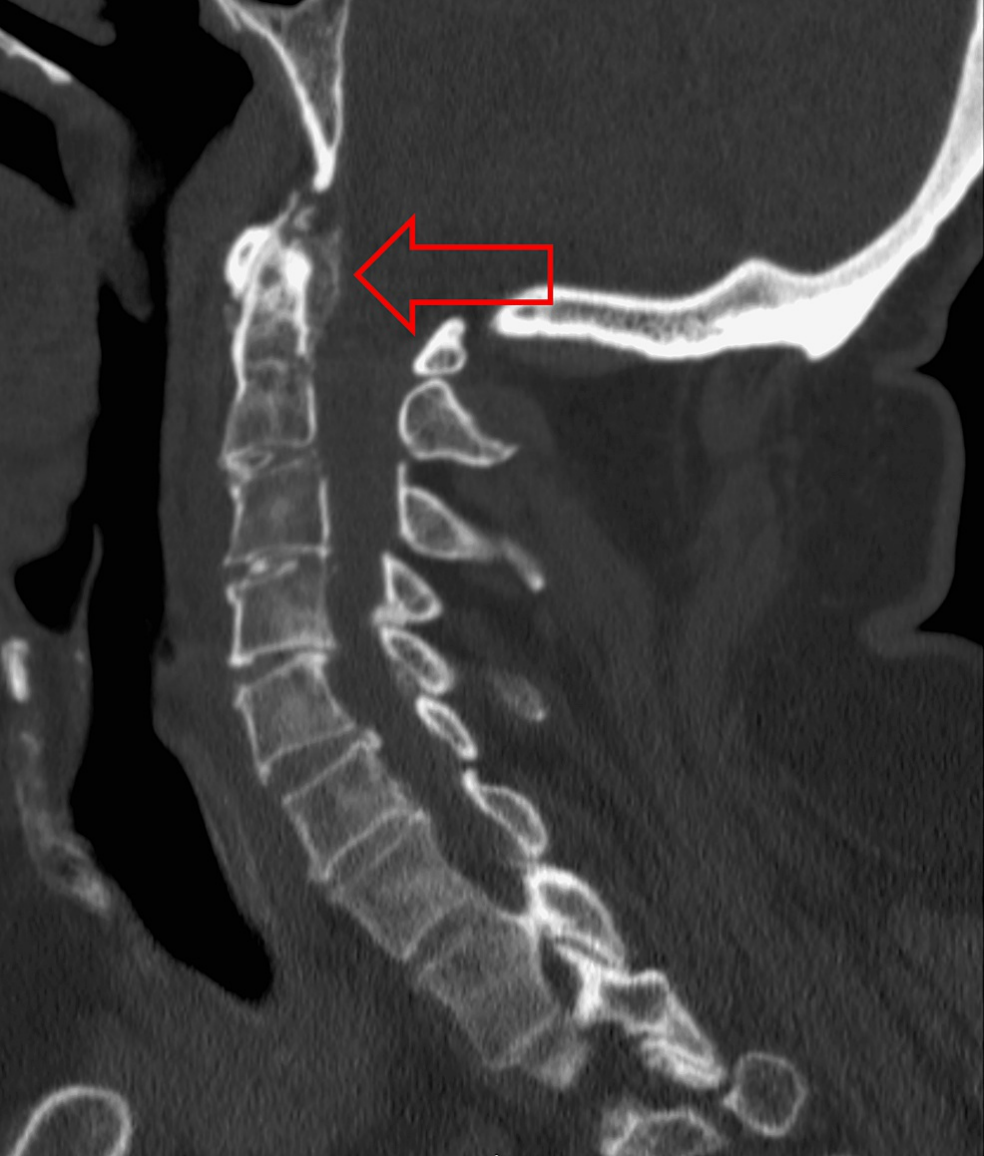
CT neck sagittal view showing area of calcification posterior to the odontoid process (red arrow).
